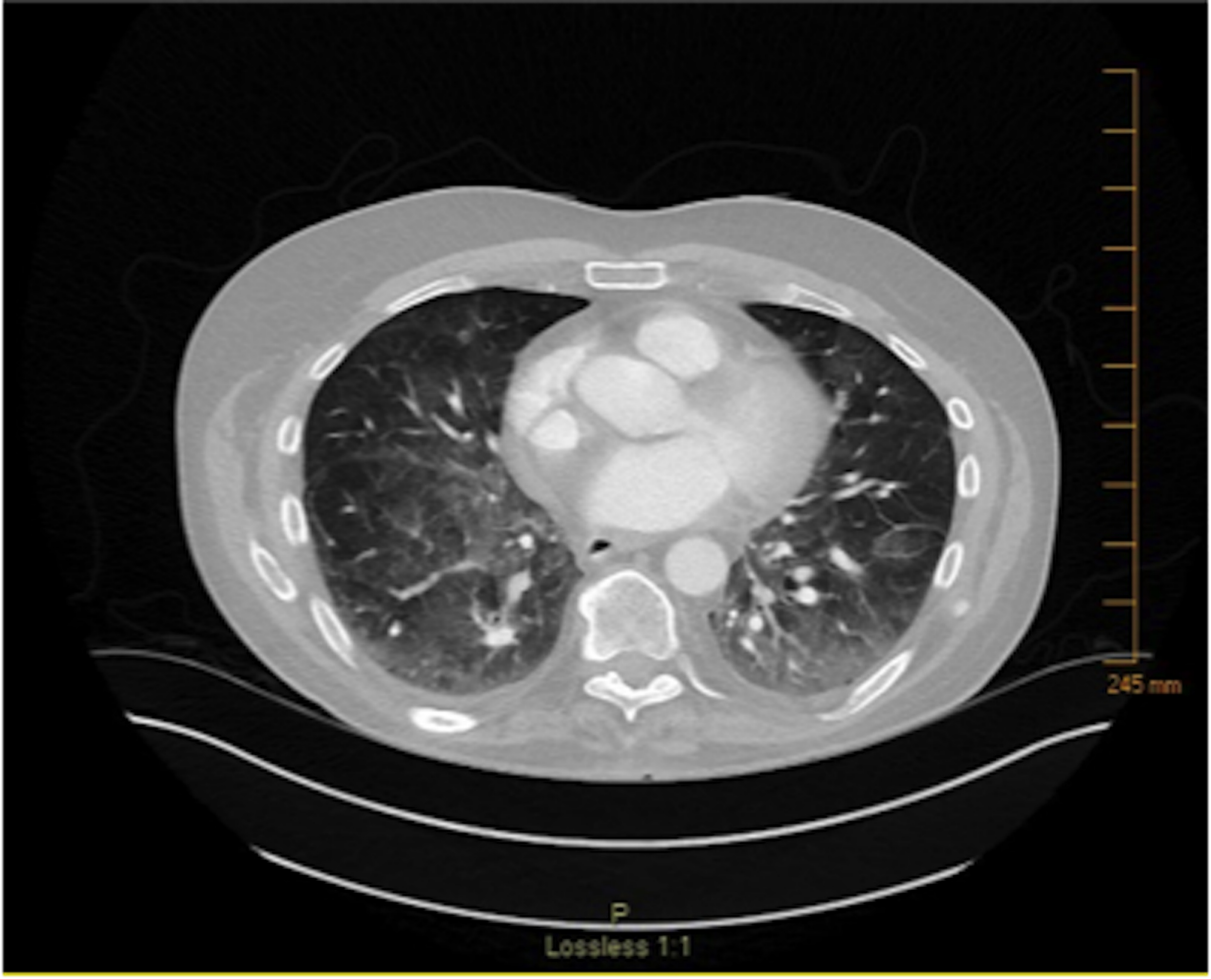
CT after course of steroids CT scan after steroid treatment, showing resolution of previously seen bilateral pleural effusions. CT: computed tomography
